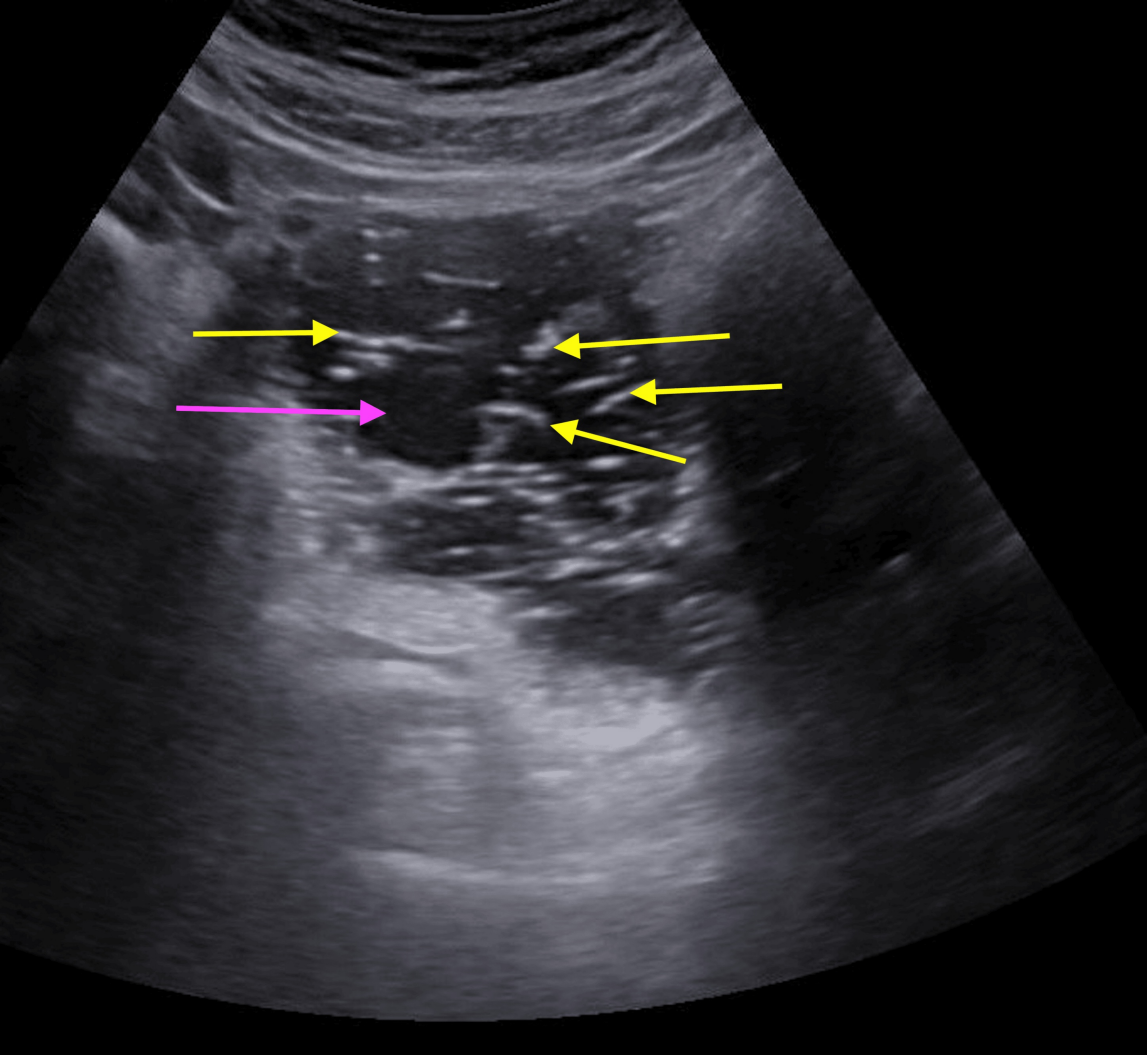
Pelvic ultrasonography (USG). The pink arrow indicates a hypoechoic lesion measuring 8.2 x 3.7 cm in the left adnexa, while the yellow arrows highlight multiple hyperechoic foci within the lesion, appearing as thin linear structures resembling hair strands.
